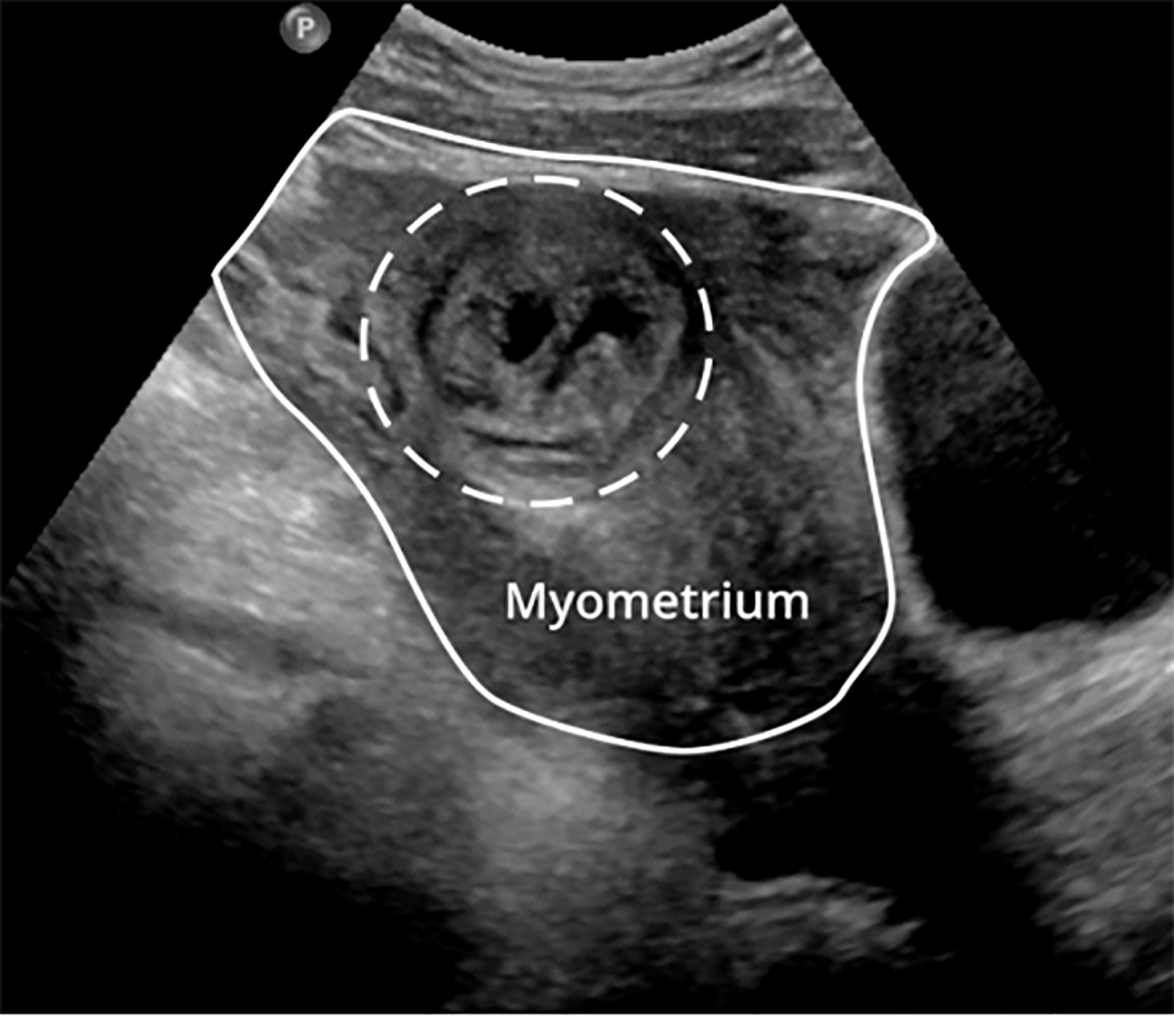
Transabdominal ultrasound demonstrating a mass in the left lateral uterus
